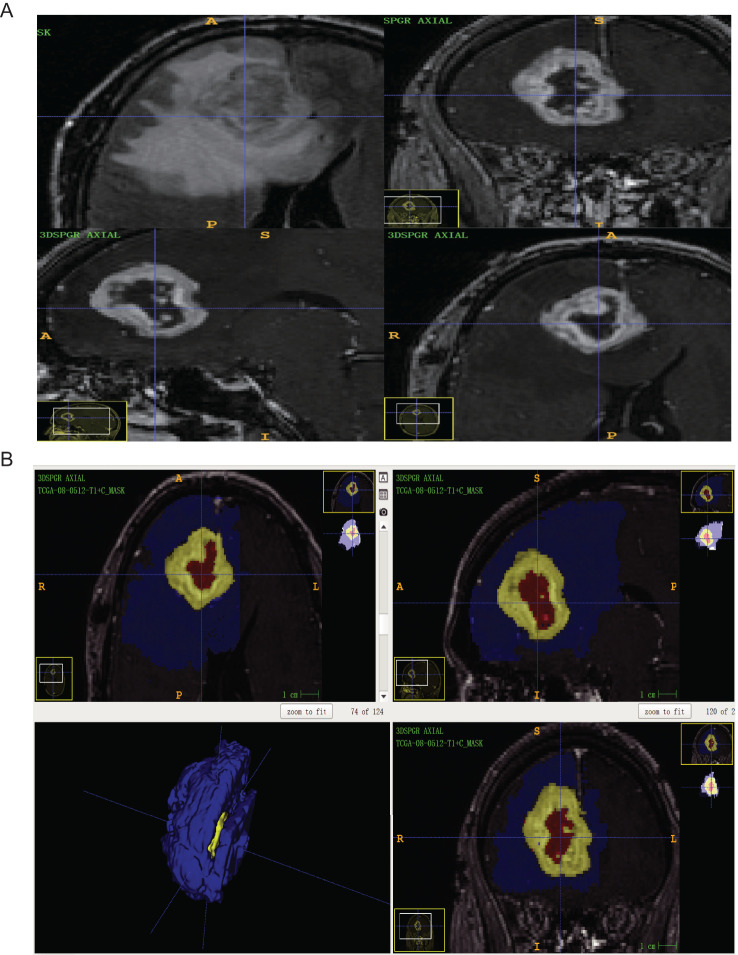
(A) The GBM patients with routine MRI scan (ID: TCGA-08-0512) were T2-FLAIR axial sequence and T1 + C three-plane, respectively; (B) ITK-snap software mapped the ROI of GBM patients with axial, sagittal, coronal, and 3D images (Blue is edema volume, yellow is tumor enhancement volume and red is tumor necrosis volume).
